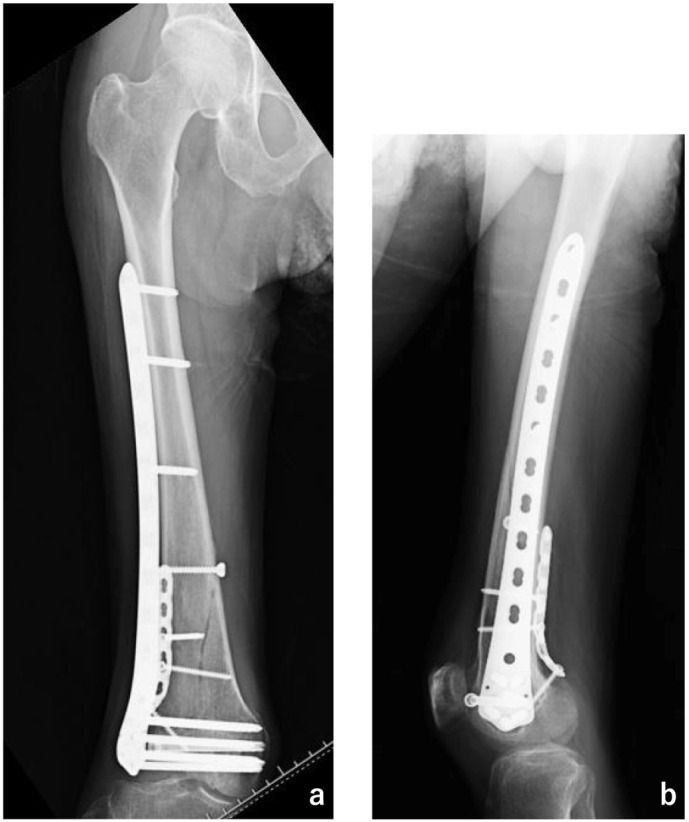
Plain X-ray at three month follow up. a: No displacement and bone union of the medial cortex was observed. b: Osteonecrosis of the lateral condyle is not evident.
